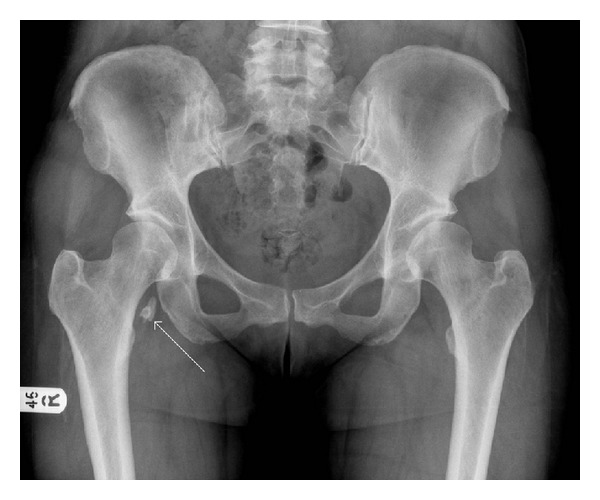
An anterior-posterior plain radiograph of the patient's hips taken 3 months prior to the repeat left psoas release surgery. There are only mild dysplastic changes present in the left hip. There is significant ossification, demonstrated by the arrow, superior to the right lesser trochanter, which is likely secondary to the patient's right psoas tenotomy undertaken 11 years ago.
